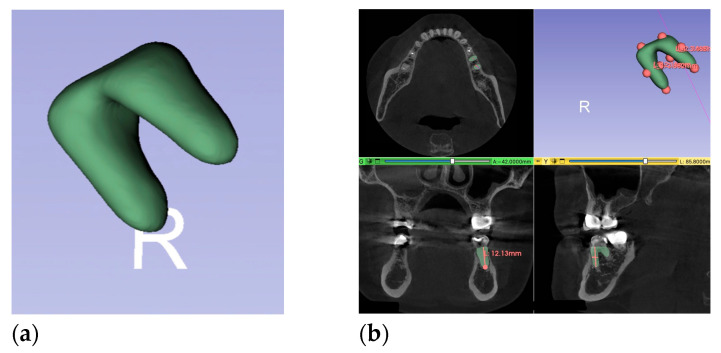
(a) tooth root from the CBCT with 3D-Slicer; (b) root in all axes in the CBCT with length, width, and depth measurements.
